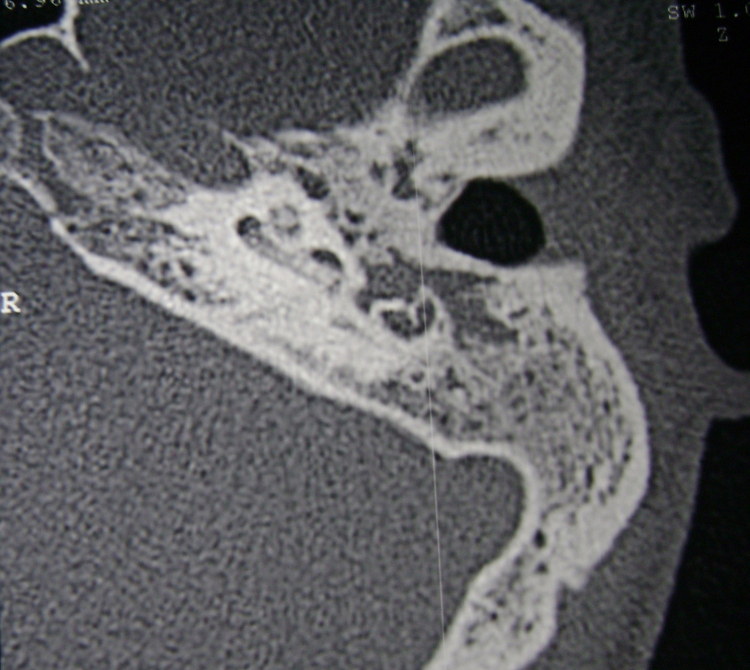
Left temporal bone CT, axial view, showing cochlear ossification. *Note*: underdeveloped mastoid filled with soft tissue-density material.
